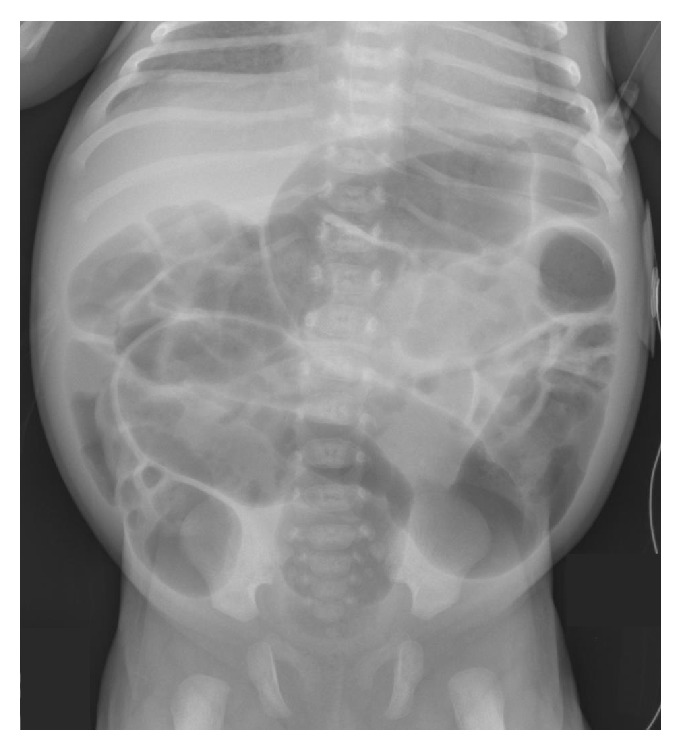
A representative abdominal radiograph obtained on HD 11 revealed gaseous distention consistent with an adynamic ileus without evidence of bowel obstruction. A paucity of air in the rectum is again seen.
